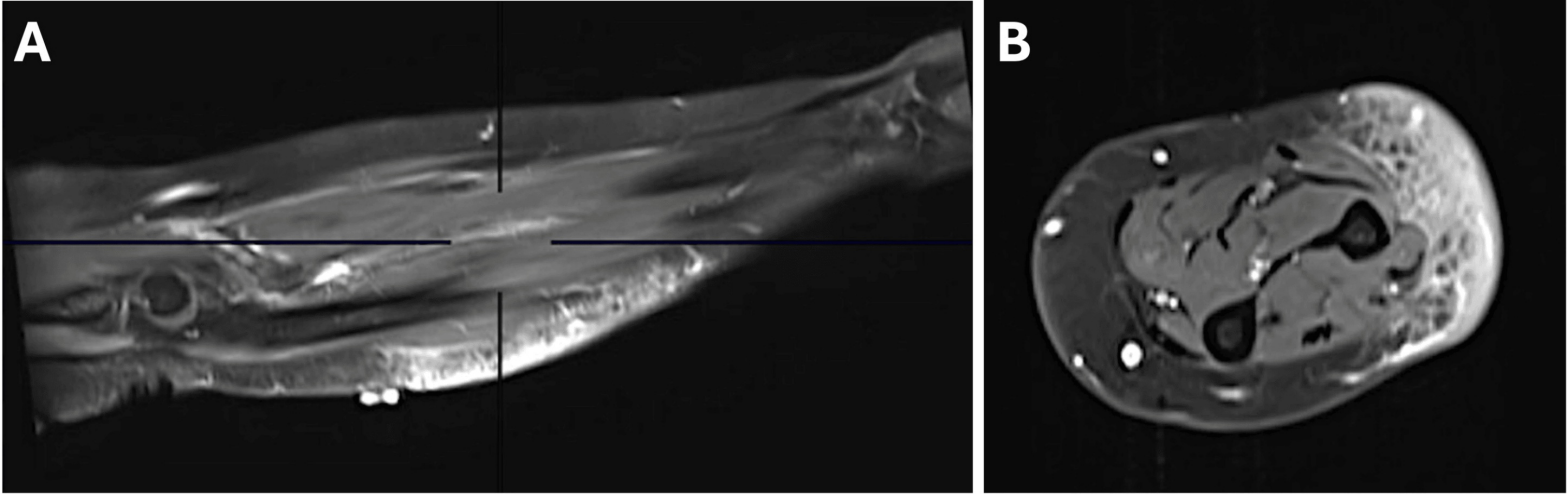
Enhancement on MRI views of the left forearm. Coronal (A) and axial (B) MRI views of the left forearm demonstrate ill-defined diffuse skin and subcutaneous adipose tissue thickening, T2 hyperintense edema, and diffuse enhancement of the ulnar forearm.
